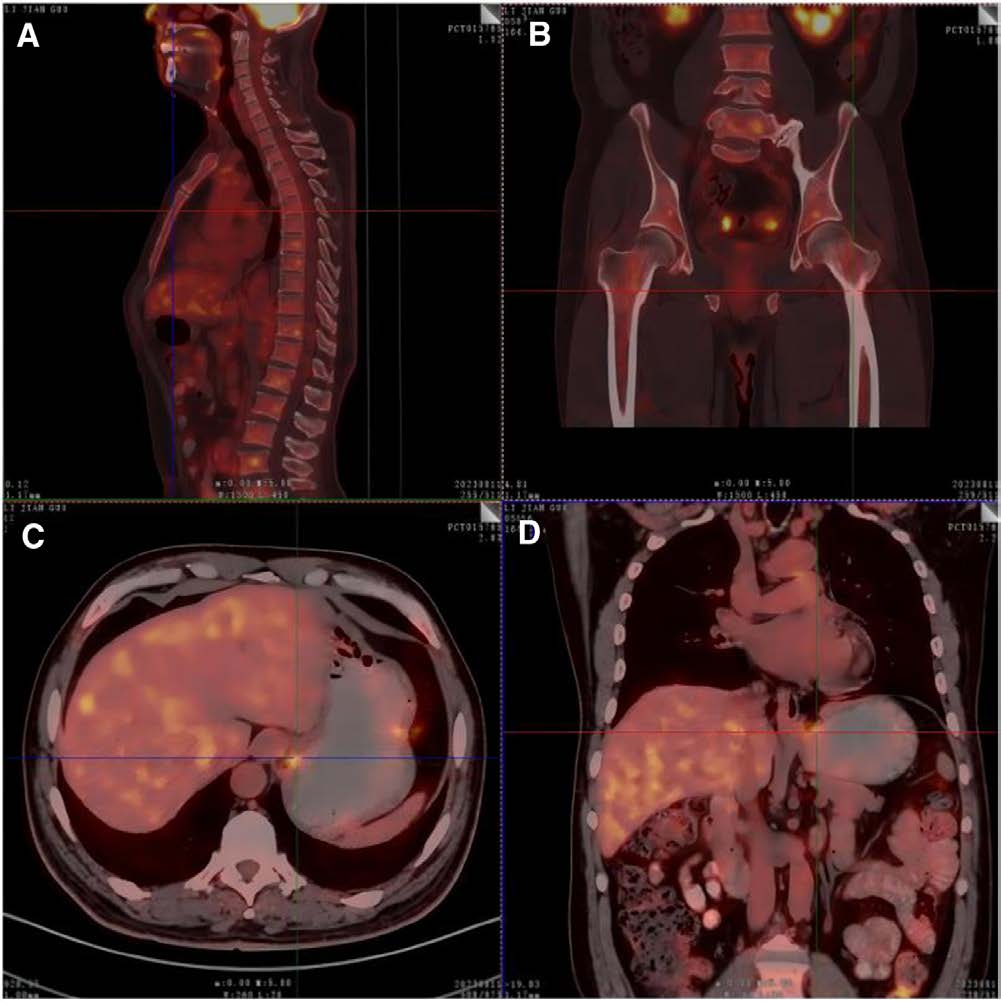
(A–D) PET-CT examination showed diffuse and uneven hypermetabolism of systemic bone marrow, accompanied by uneven bone marrow density. An irregular mass at the gastroesophageal junction, accompanied by thickening and increased metabolism. PET-CT = positron emission tomography-computed tomography.
